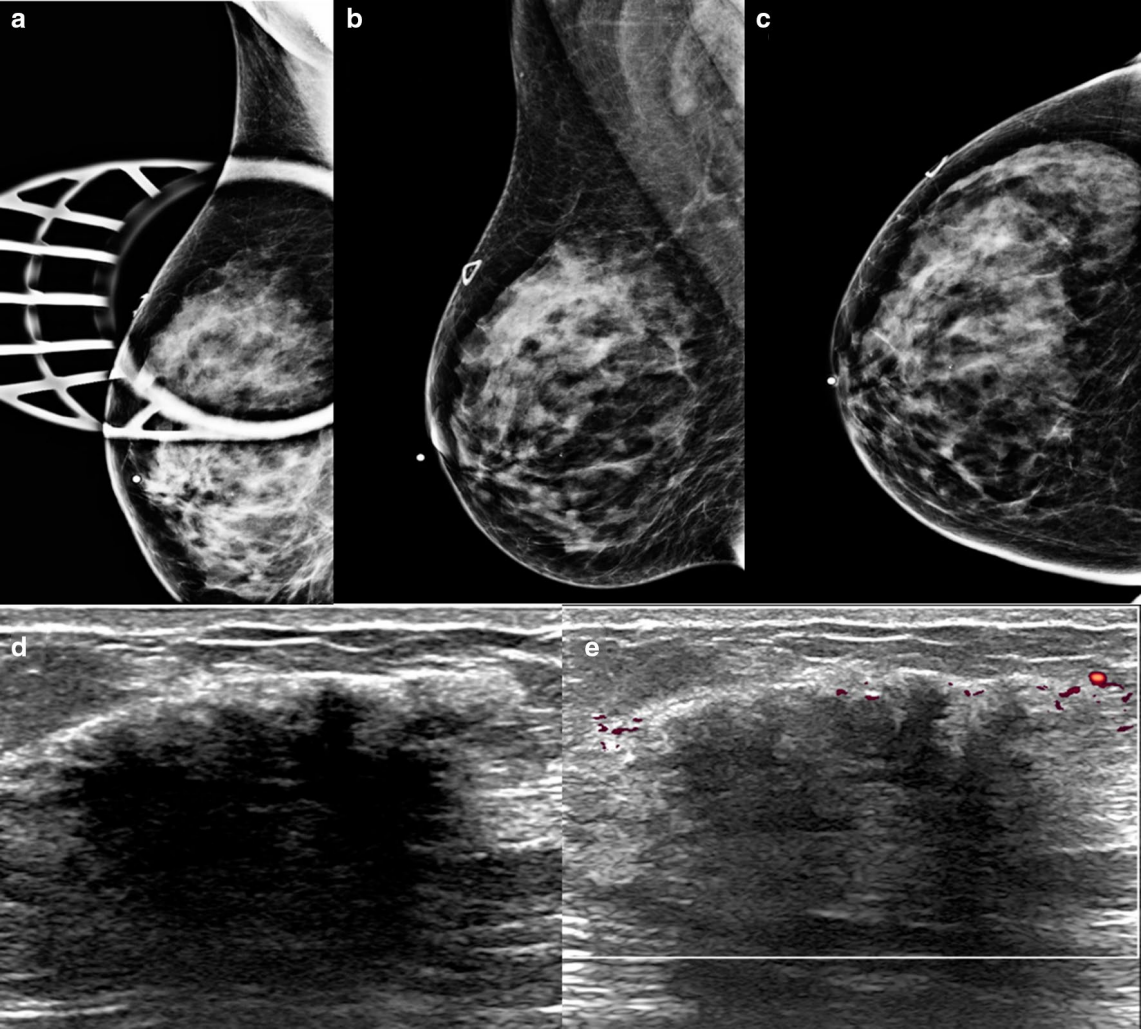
Stromal fibrosis. A 48-year-old female presented with a palpable breast mass. Spot compression tangential (a), mediolateral (b), and craniocaudal (c) mammographic views did not show a corresponding abnormality. Grayscale (d) and power Doppler (f) ultrasound images reveal an irregular mass with posterior acoustic shadowing corresponding to the palpable area. Core needle biopsy of the mass showed stromal fibrosis without evidence of malignancy
